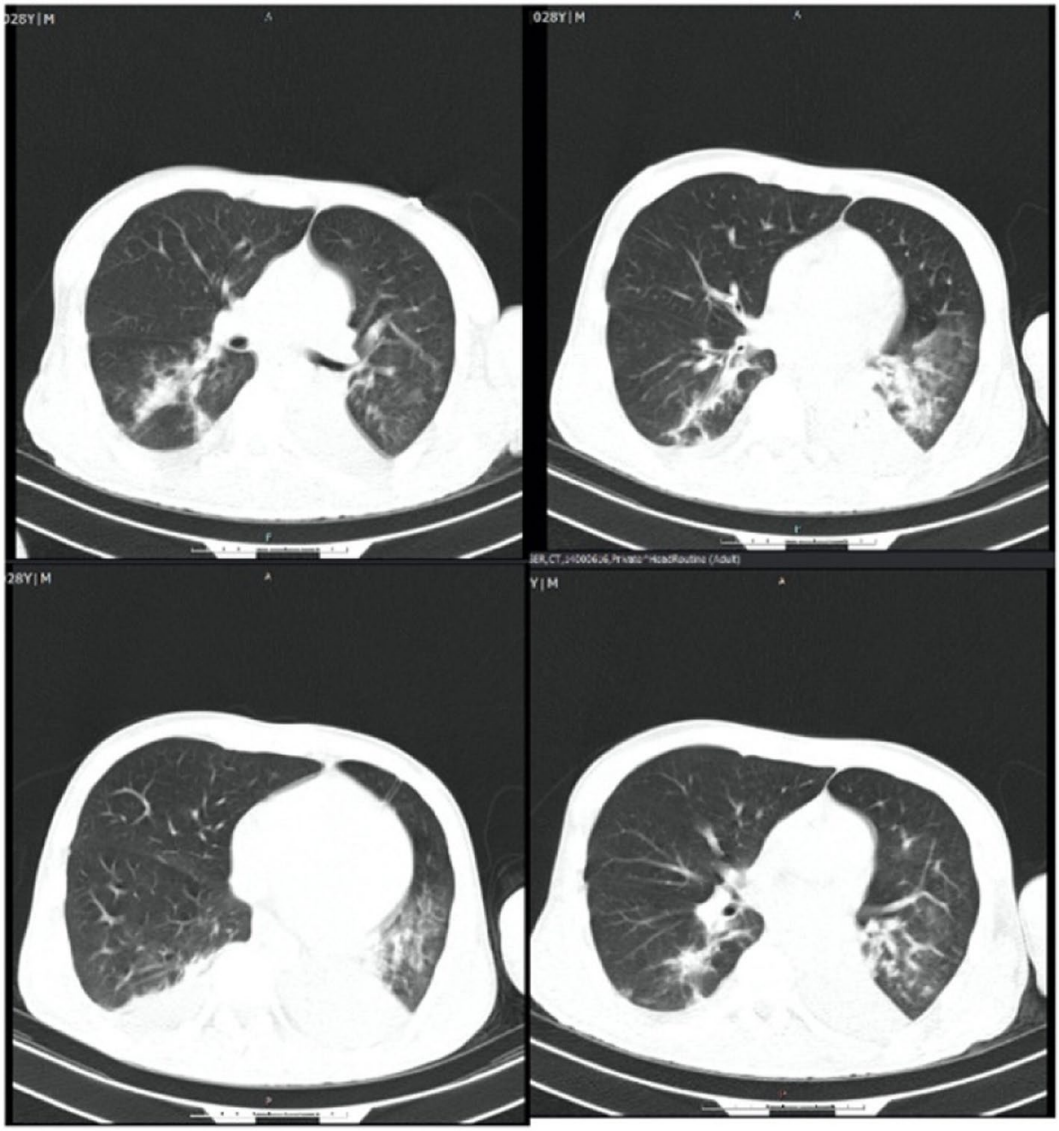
Lung computed tomography scan of the patient
